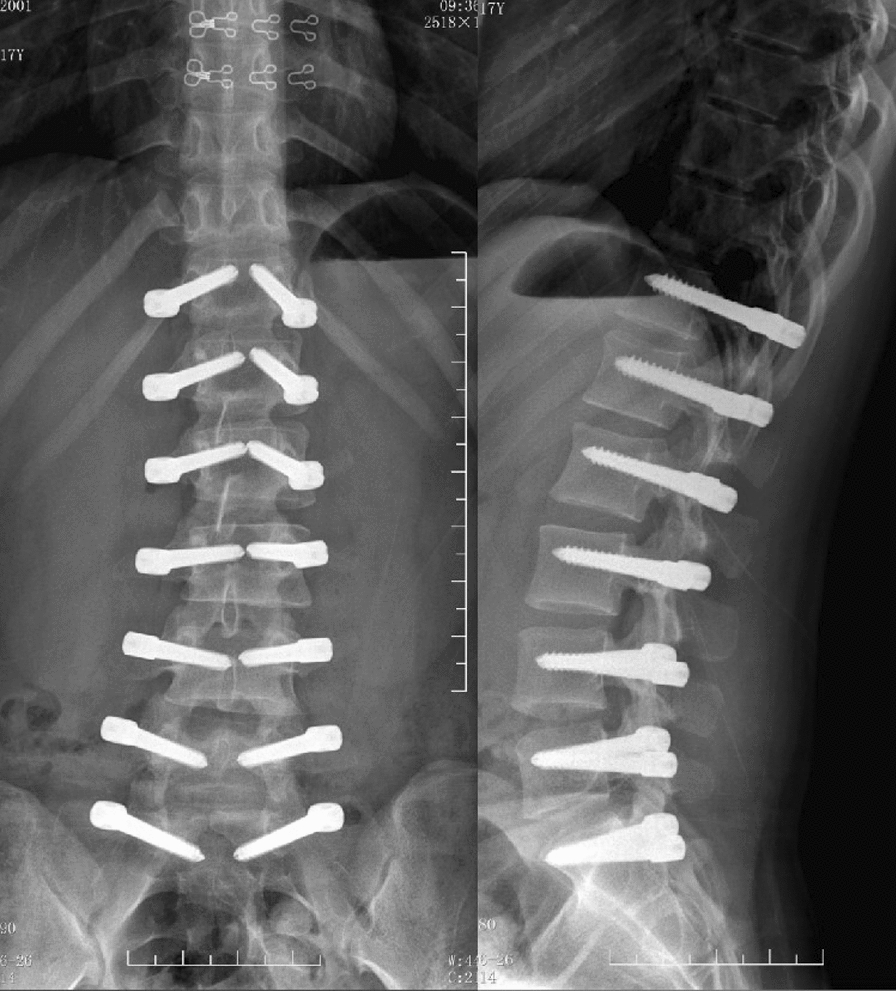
After surgery, the X-ray showed that the lumbar curvature was 3.6°, and the lumbosacral curvature was 0.4°
